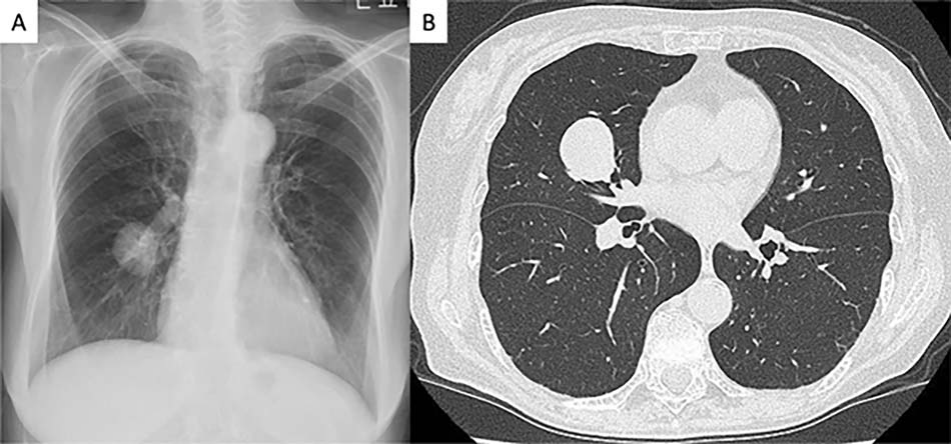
Radiologic findings (A) Chest radiograph showed a round mass in the right middle lung field. (B) Computed tomography image showing a 28-mm nodule in the middle lobe of the right lung.
